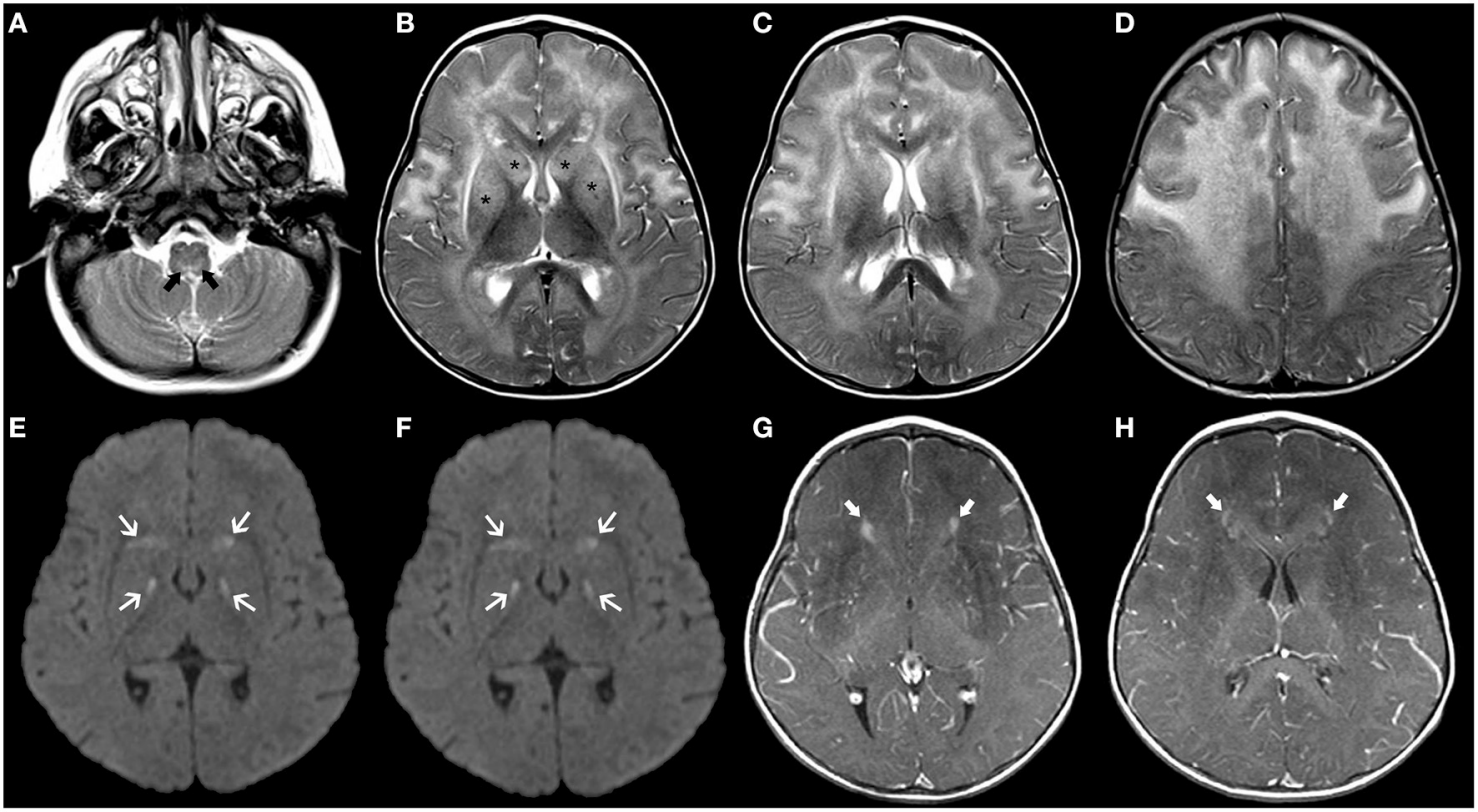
Brain MRI findings of Alexander Disease (infantile form). Axial T2WI (A–D) of a 1.2-years-old girl exhibit diffuse, symmetric, and confluent areas of white-matter hyperintensity with a postero-anterior gradient. Also, note areas of hyperintensity in the medulla oblongata (thick black arrows) as well as in the caudate nuclei and putamina (asterisks). Axial b1000 images (E,F) show focal areas of mild symmetric hyperintensity involving the globus pallidus and the head of the caudate bilaterally (white arrows). There was corresponding hypointensity in the ADC maps (not shown) in keeping with restricted diffusion. Axial post-gadolinium T1WI (G,H) depict small “caps” of contrast enhancement around the frontal horns bilaterally (thick white arrows).
